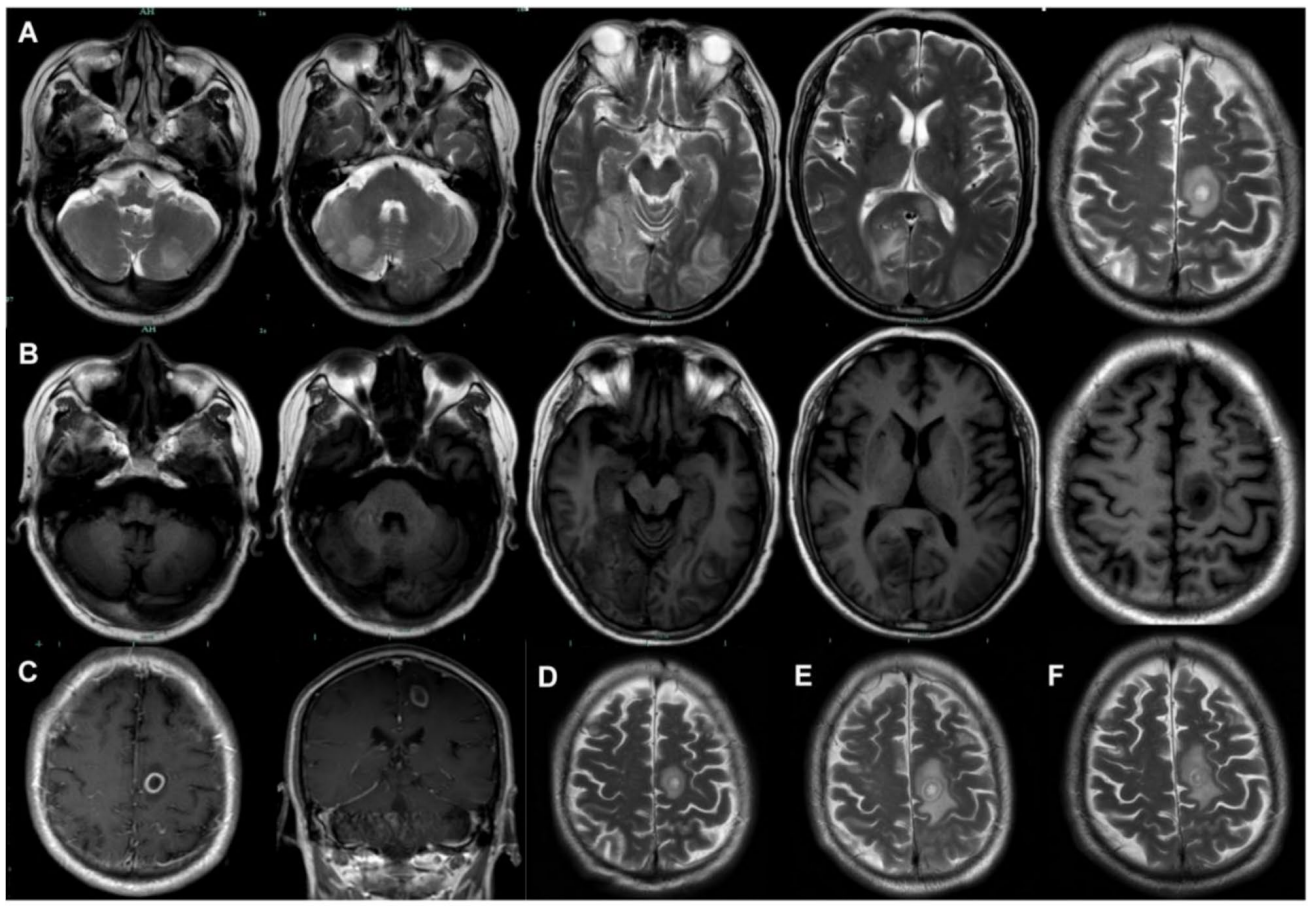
Multiple signal abnormalities in the bilateral cerebellum, frontal, temporal, parietal, occipital lobes, and corpus callosum. (A) T2-weighted imaging. (B) T1-weighted imaging. (C) T1-weighted contrast-enhanced imaging: the abscess with a diameter of 13.1 mm, surrounded by a hyperintense ring with peripheral patchy hypointensity. (D-F) Axial T2-propeller imaging: the abscess following stroke on day 0, day 21, day 35
